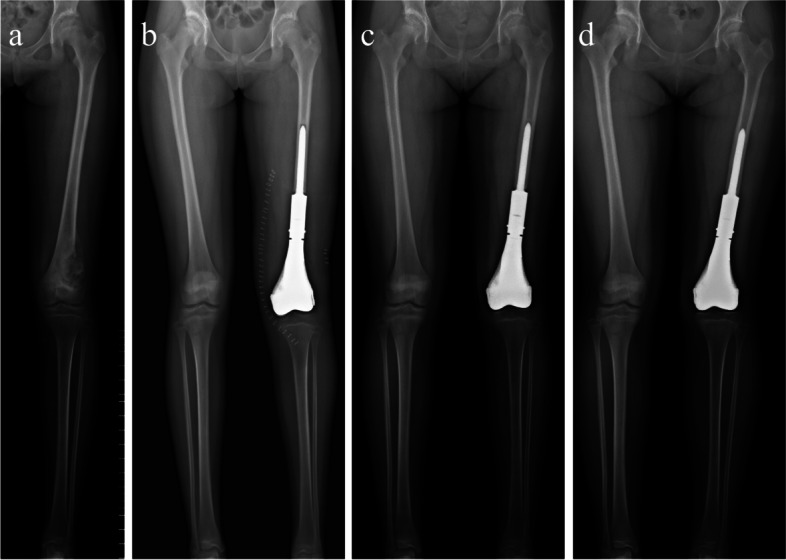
The radiograph of an eleven-year-old girl with osteosarcoma in the distal femur (case 7). a Preoperative radiograph showing the distal femoral physis involved in the tumor; b Radiograph after surgery showed that the patient underwent hemiarthroplasty with the second-generation prosthesis following tumor resection, and the prosthesis is 1 cm longer than the length of resection; c Eight months postoperative radiograph; d Radiograph 18 months after surgery showing an LLD of 0.8 cm. LLD, limb length discrepancy
